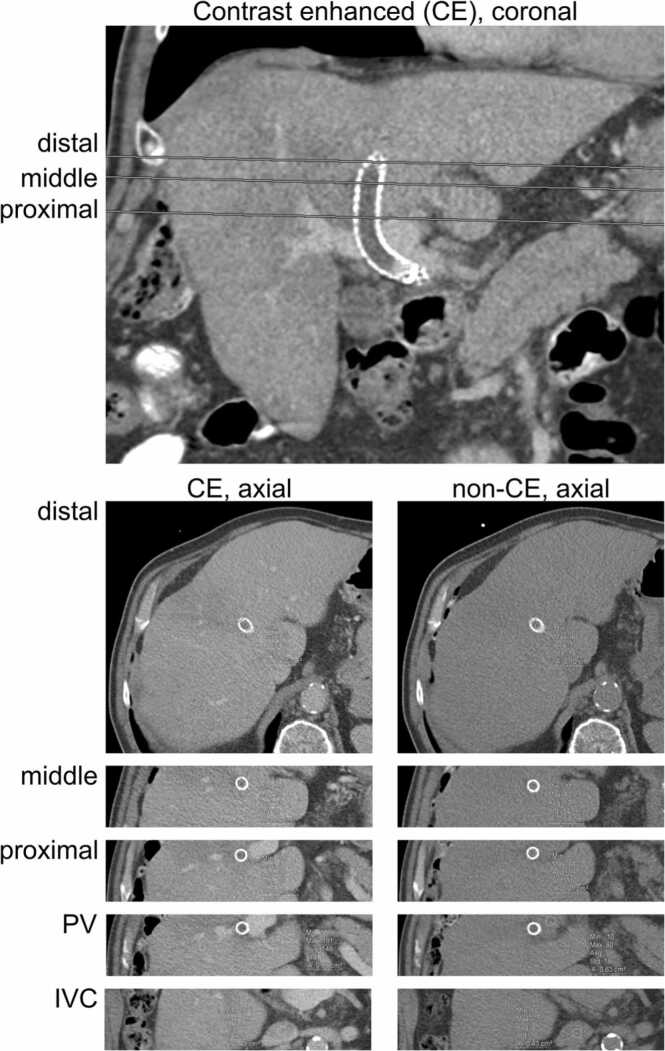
Example of representative region of interest (ROI) placement. Contrast-enhanced (CE) and unenhanced computed tomography examination of the abdomen of a patient with a thrombus within the lumen of the transjugular intrahepatic portosystemic shunt (TIPS). The coronal plane depicts the respective levels of axial ROI-placement within the TIPS lumen. In this patient, all three axial planes represented areas of highest suspicion of in-TIPS thrombosis, therefore an additional ROI of highest suspicion is not depicted. Respective intraluminal regions used to measure the portal vein (PV) and inferior vena cava (IVC) are depicted. Quantitative measurements consisted of minimum, maximum, average, standard deviation and area.
